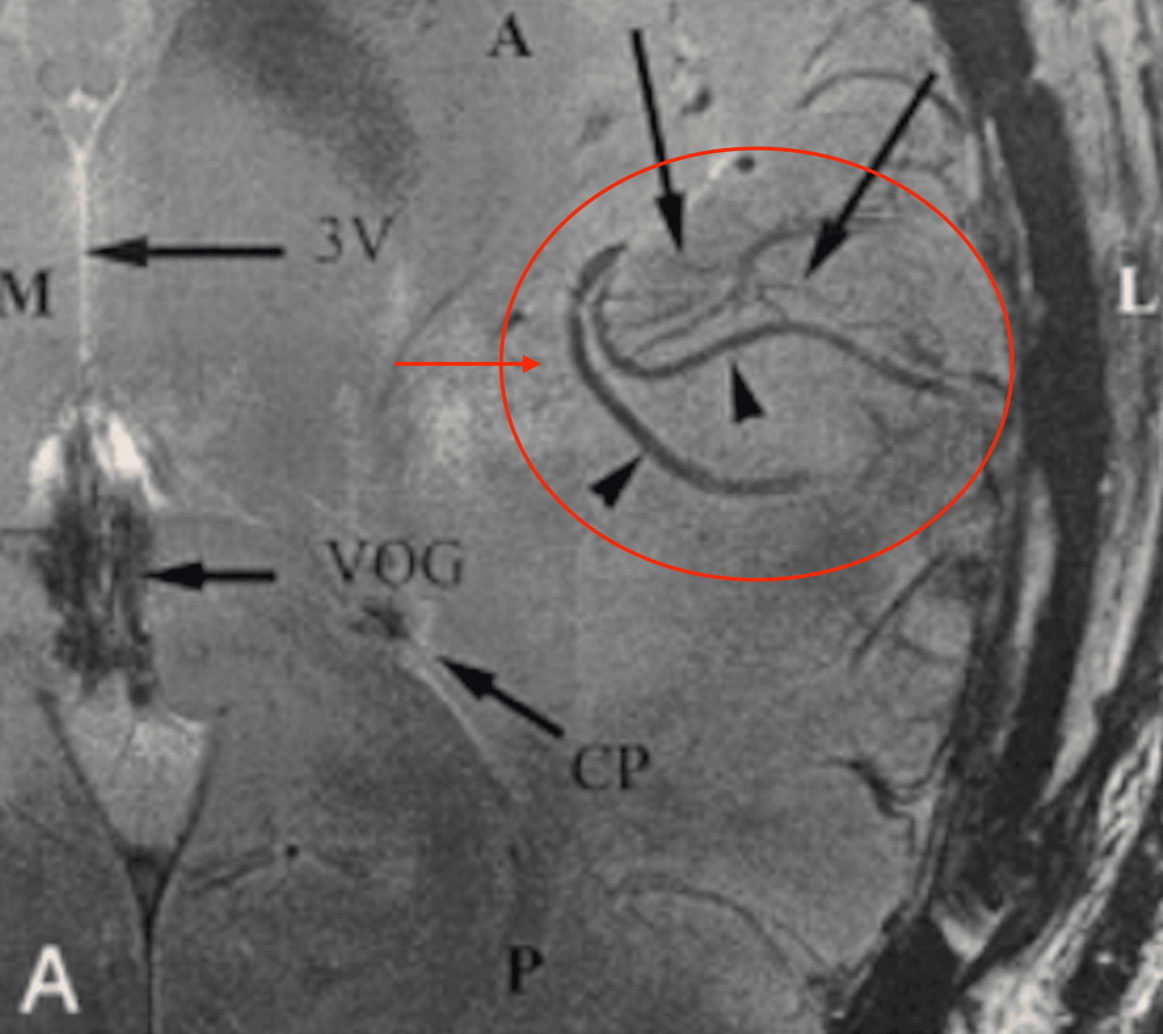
Increased angiogenesis seen in patients with glioblastoma using a standard MRI procedure CP: choroid plexus; VOG: vein of Galen; 3V: third ventricle. Image Source: Christoforidis et al., 2002 [8]; Used with permission from the American Society of Neuroradiology.
